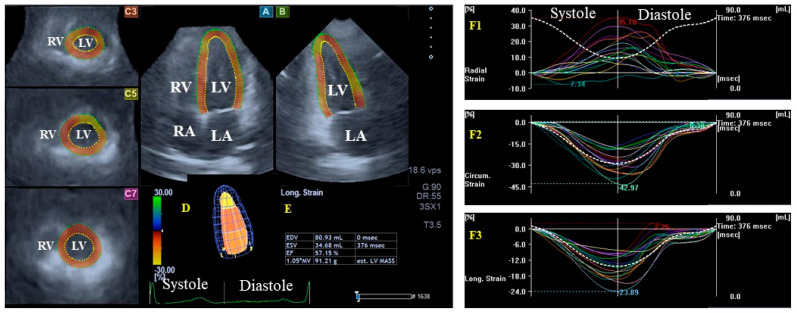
Assessment of the left ventricular (LV) strains by three-dimensional (3D) speckle-tracking echocardiography. Apical longitudinal four-chamber (A) and two-chamber (B) views and short-axis views at the basal (C3), midventricular (C5), and apical levels (C7) of the LV are presented together with a 3D cast of the LV (D) and the LV volumetric data calculated (E). Curves of time—global radial (F1), circumferential (F2), and longitudinal (F3) LV strains (colored lines) and time—change in the LV volume (dashed white line) during the cardiac cycle are shown together. Abbreviations: LA = left atrium; LV = left ventricle; RA = right atrium; RV = right ventricle; EDV = end-diastolic volume; ESV = end-systolic volume; EF = ejection fraction.
